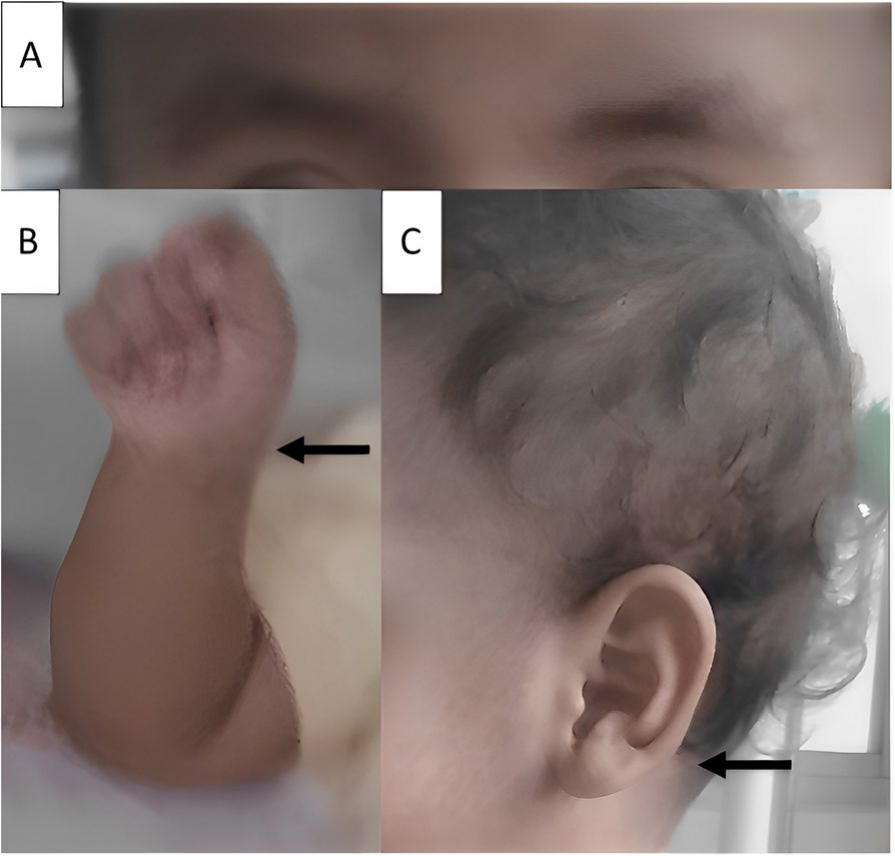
Photograph of the patient showing facial appearance of Costello syndrome. A Thick eyebrows. B Ulnar deviation of hands. C Low-set ear, large pinnaThe musculoskeletal evaluation revealed a short neck, hypotonia, increased anteroposterior chest diameter, broad distal phalanges, ulnar deviation of the hands (Fig. 2B), and pectus carinatum. Skin assessment based on epithelial alterations revealed loose skin of the hands and feet, curly hair, and deep palmar and plantar folds.At 20 months of age (14 days before admission), the patient presented with a typical clinical condition of viral illness and received symptomatic treatment for loose stools and a cold. His mother stated that seven days before admission, the patient presented with altered visual acuity (accidentally bumping into walls and objects), and five days before admission, she observed marked hypotonia. The patient was referred to a pediatric neurologist and received treatment, though he showed no improvement.Upon hospital admission, the patient presented generalized seizures and aspiration pneumonia, and was consequently admitted to the pediatric intensive care unit. The brain magnetic resonance imaging revealed symmetrical lesions of the infra- and supratentorial white matter in both cerebral hemispheres: the centra semiovale, periventricular white matter and the corpus callosum (front and posterior part). No morphological or brain parenchymal signal-intensity abnormalities were detected nor any alterations in the cerebellar hemispheres, cerebellopontine angles, thalamus, basal ganglia, pituitary gland, eyeballs, or optic nerves (see Fig. 3).The patient was diagnosed with cerebral leukodystrophy of unknown etiology. Etiology tests for leukodystrophy were not possible due to the negative prognosis and progressive deterioration and the patient died.Fig. 3 Fig3:
